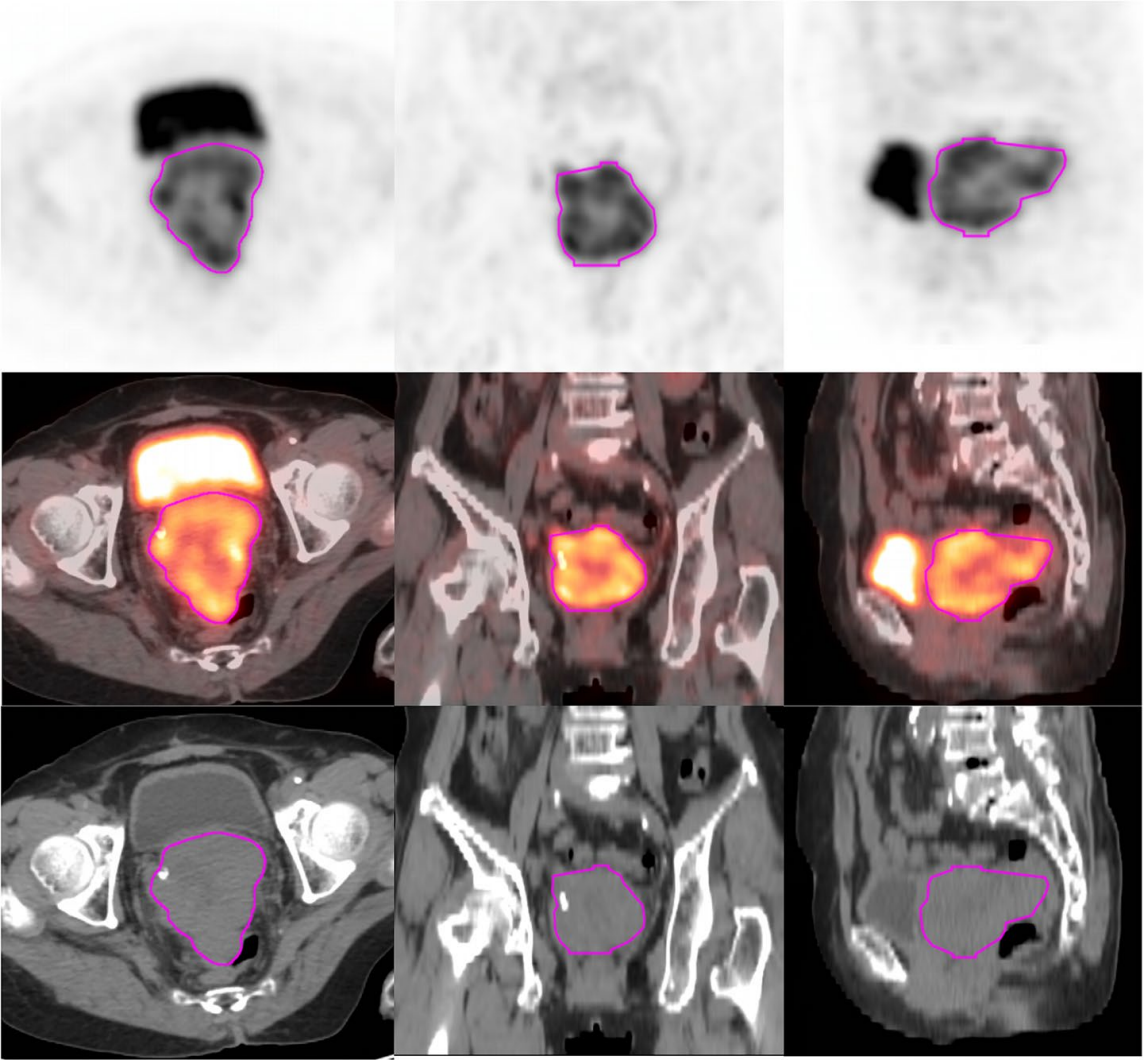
Representative example of the regions of interest (ROI) segmentation on axial, coronal, and sagittal PET/CT
